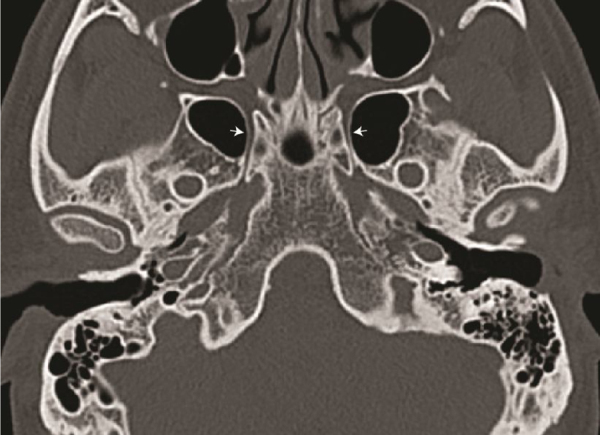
Axial computed tomography scan slide showing the vidian canal (white arrow) for length measurement.
